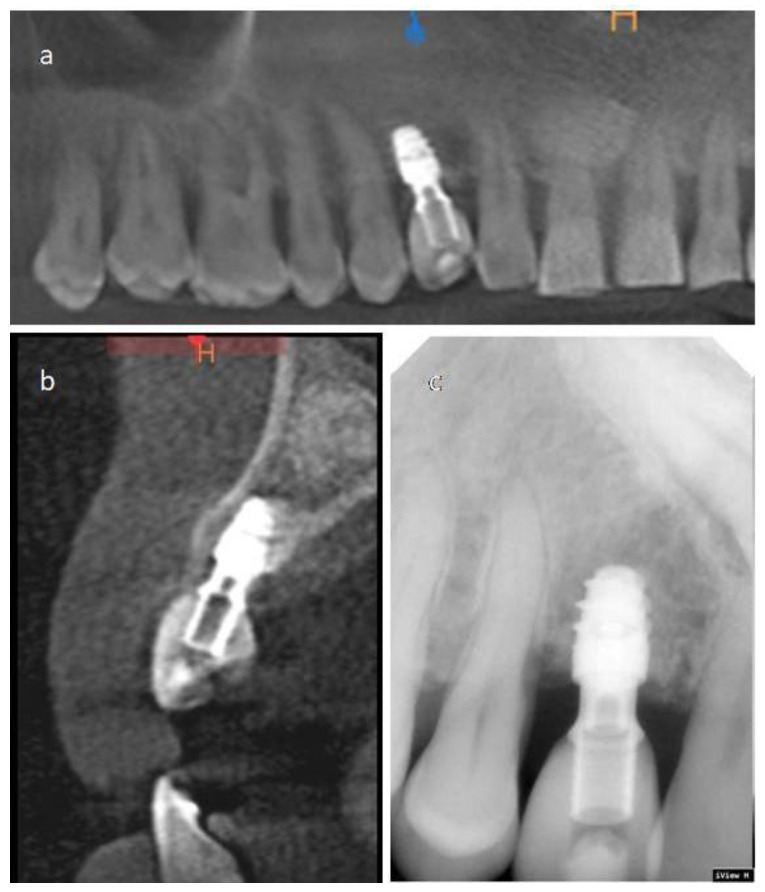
Case 1 last follow-up (3 years after the surgery). (a) CT-Scan image analogous to panoramic radiography of the first quadrant. (b) Sagittal section shows the dental implant. (c) Periapical radiograph.
